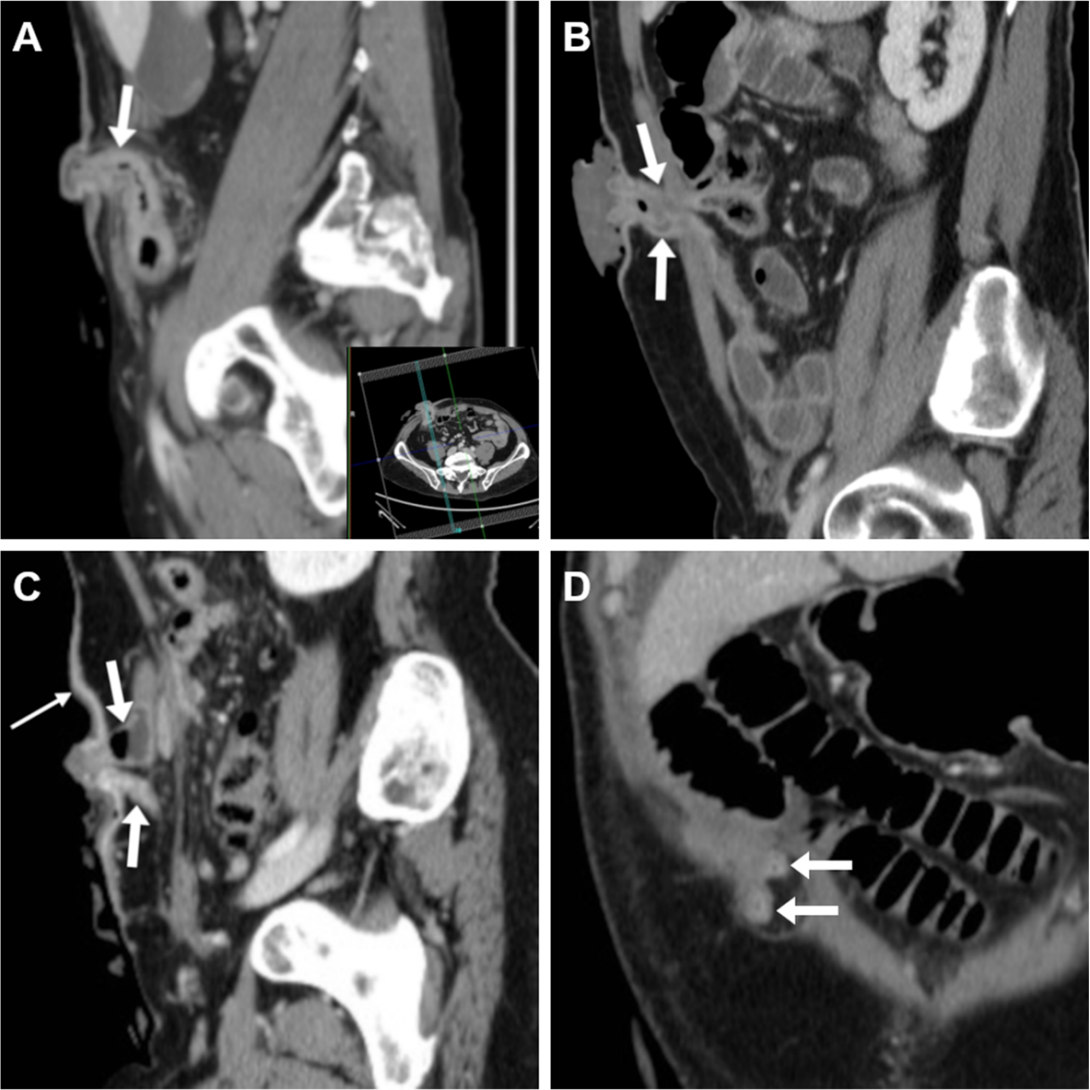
CT reconstruction technique and expected findings of ileostomies. a Focused oblique-sagittal (note lateral obliquity in inset) image of a normal end ileostomy with uniform mural thickness and enhancement of the stomal tract (arrow), mild protrusion at external orifice. b oblique-sagittal images of a loop ileostomy identify non-thickened, collapsed efferent and afferent limbs (arrows). Note fluid flowing at the external orifice indicating stomal patency. c, d A typical defunctioning loop ileostomy with two limbs (arrows) on sagittal (c) viewing and coronal (d) appearance. Note mild thickening and hyperenhancement of peristomal skin in c (compared to a and b) consistent with cutaneous inflammation at physical examination
